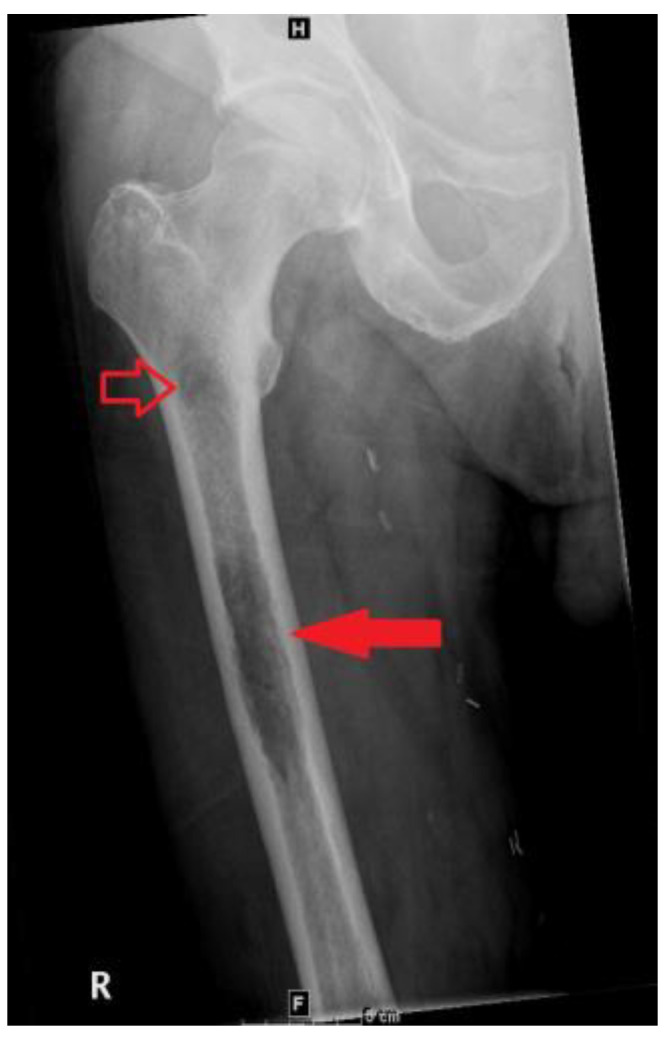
Frontal view radiograph of the right femur demonstrating a 10 cm in length lytic lesion of the proximal half of the femoral diaphysis (solid arrow), with a smaller lesion more proximally (open arrow).
